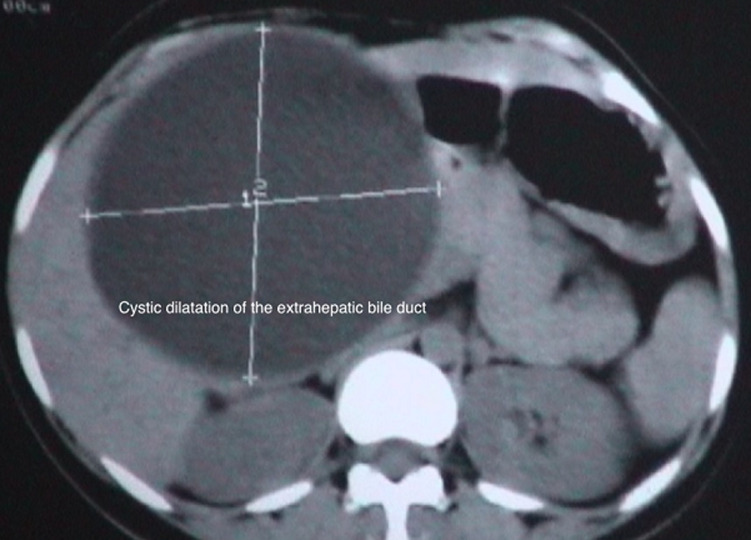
plain CT abdomen showing a cystic space-occupying lesion in the region of the head of the pancreas displacing the transverse colon and extending till the subhepatic space
